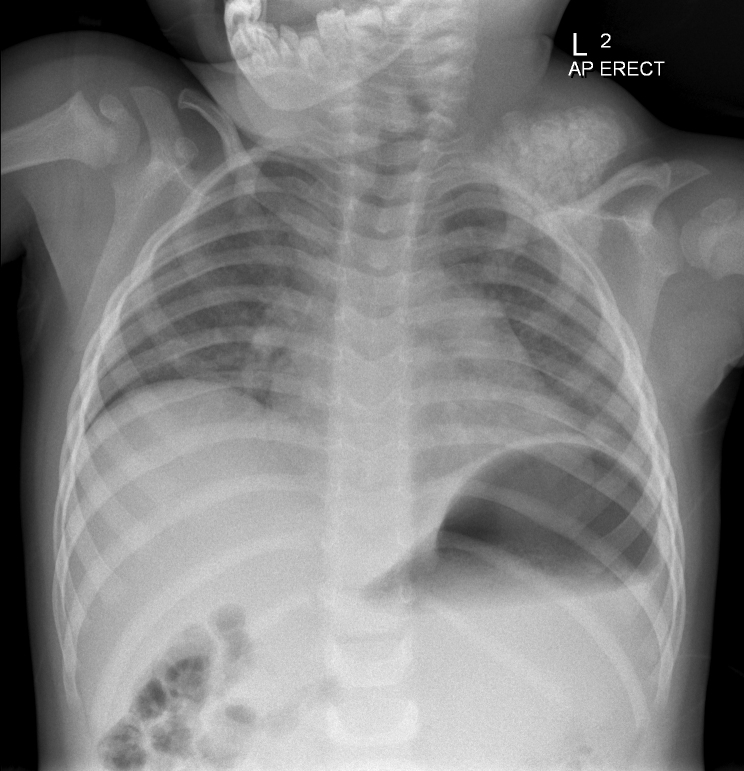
Admission chest X-ray showing a large soft-tissue mass containing heterogeneous calcification in the region of the left shoulder joint.
